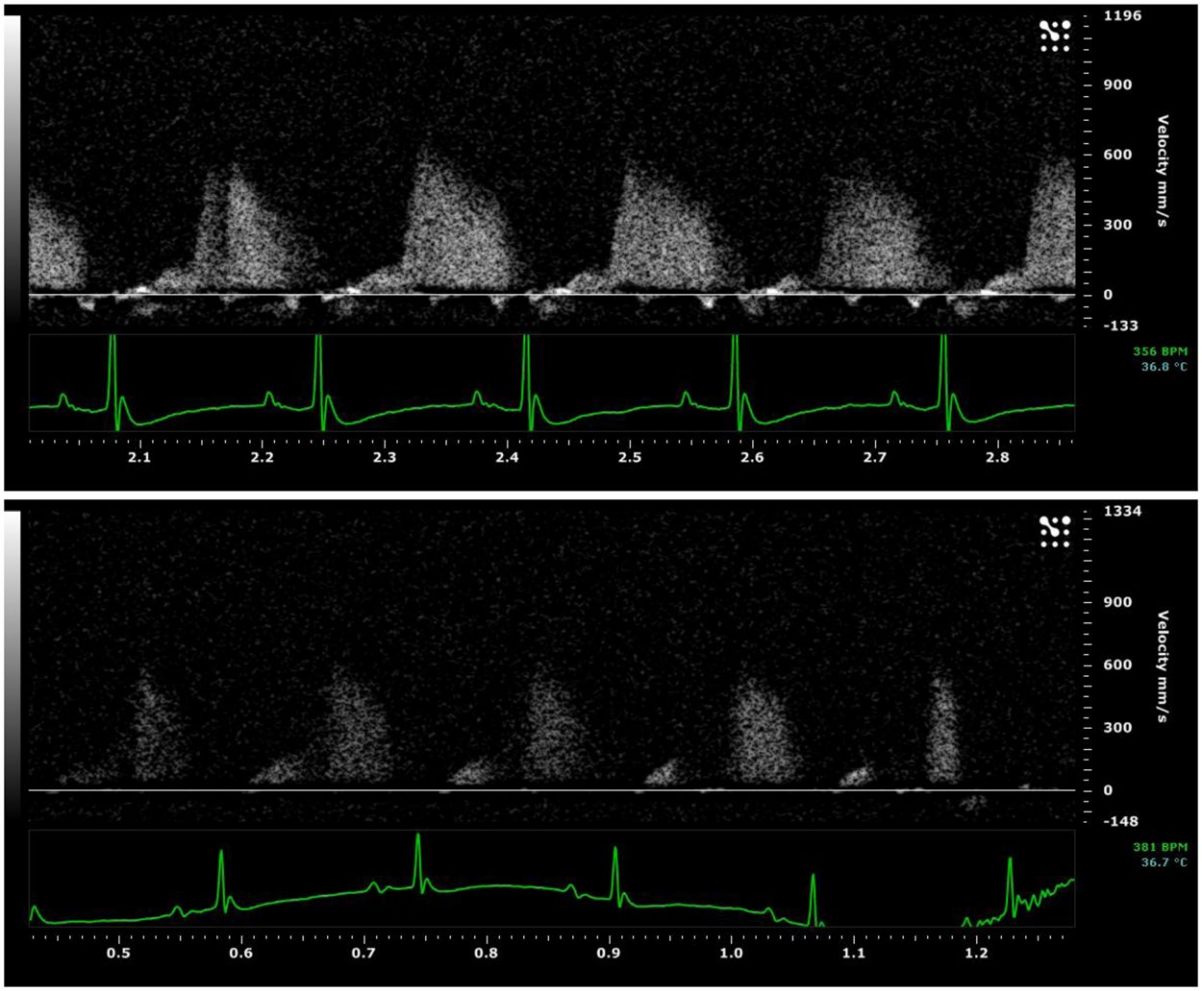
Images of two separate PW Doppler CFP recordings. The complete and expected biphasic pattern is present in the top window compared to the bottom window where a portion of the biphasic pattern is shadowed.
